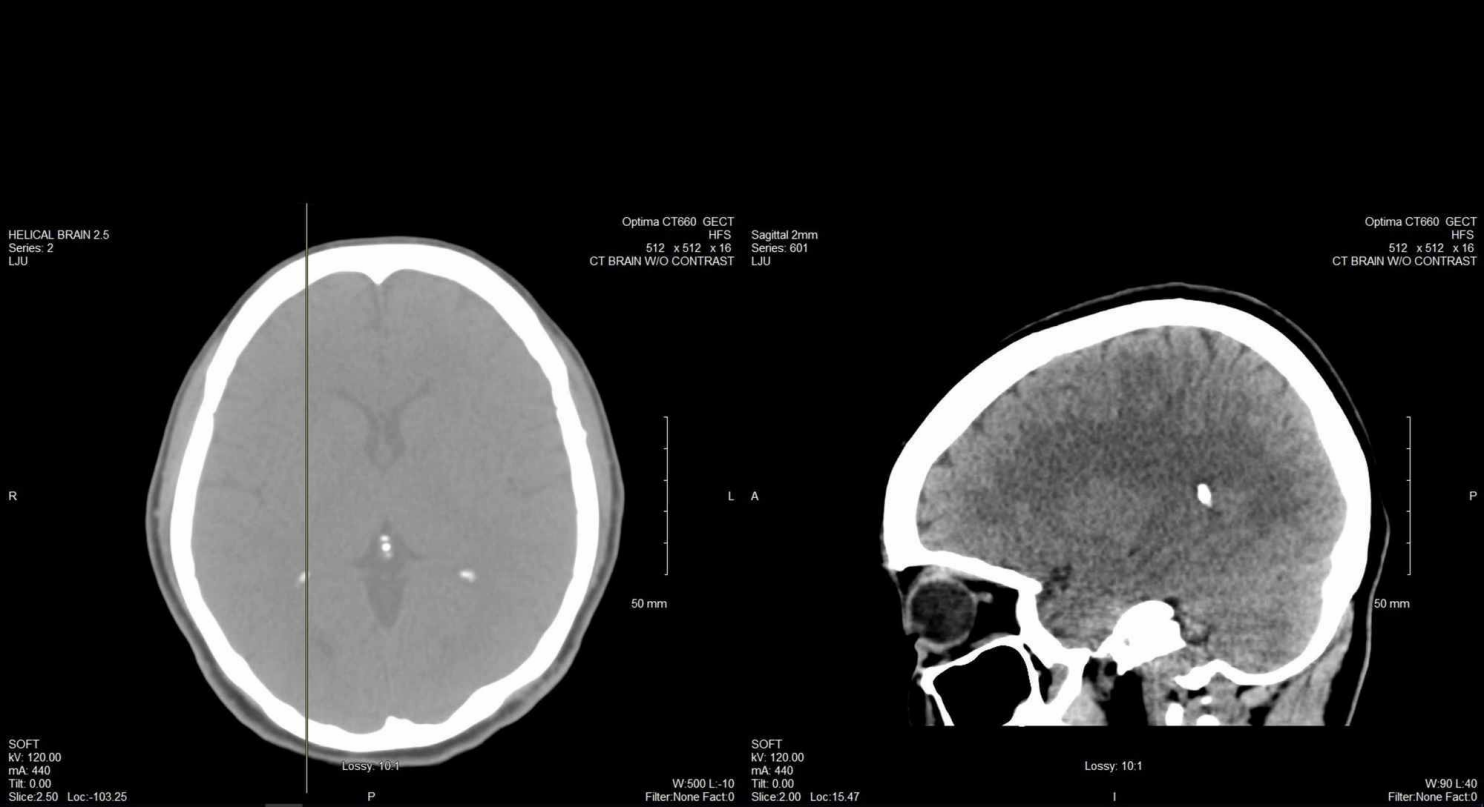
Axial and sagittal views of CT brain without contrast, highlighting the periventricular white matter hypodensities.
